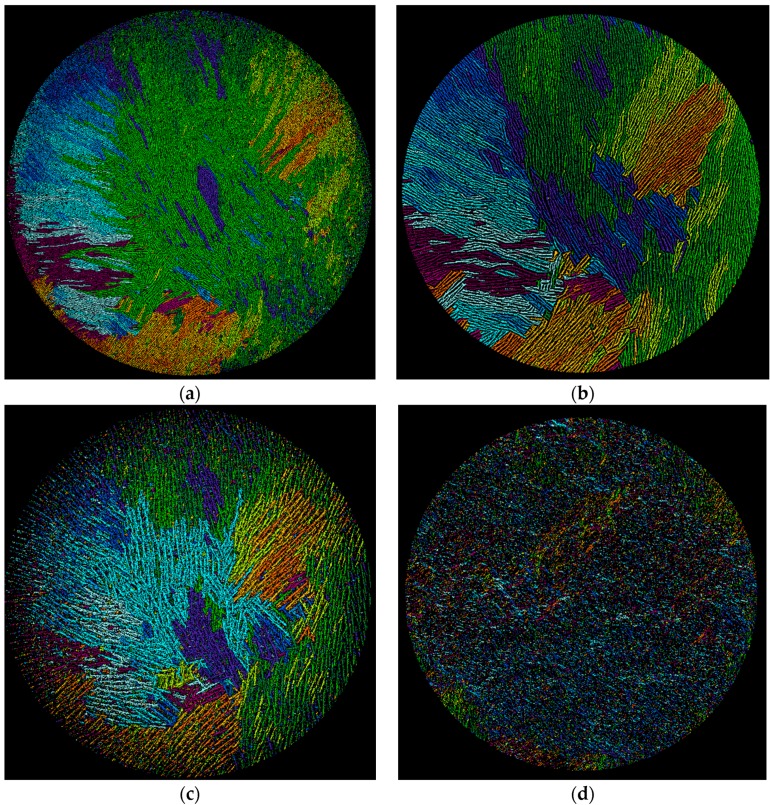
Image-analyzed X-ray micro-computed tomographs of images in Figure 5a–d to reveal orientation of domains at different heights, h, within monolith, h (cf. Figure 1a and Figure 5). (a) φ_v_ = 0.128, h = 0.90, pores image; (b) φ_v_ = 0.128, h = 0.5, lamellae image; (c) φ_v_ = 0.128, h = 0.18, pores image; (d) φ_v_ = 0.032, h = 0.9, lamellae image.
